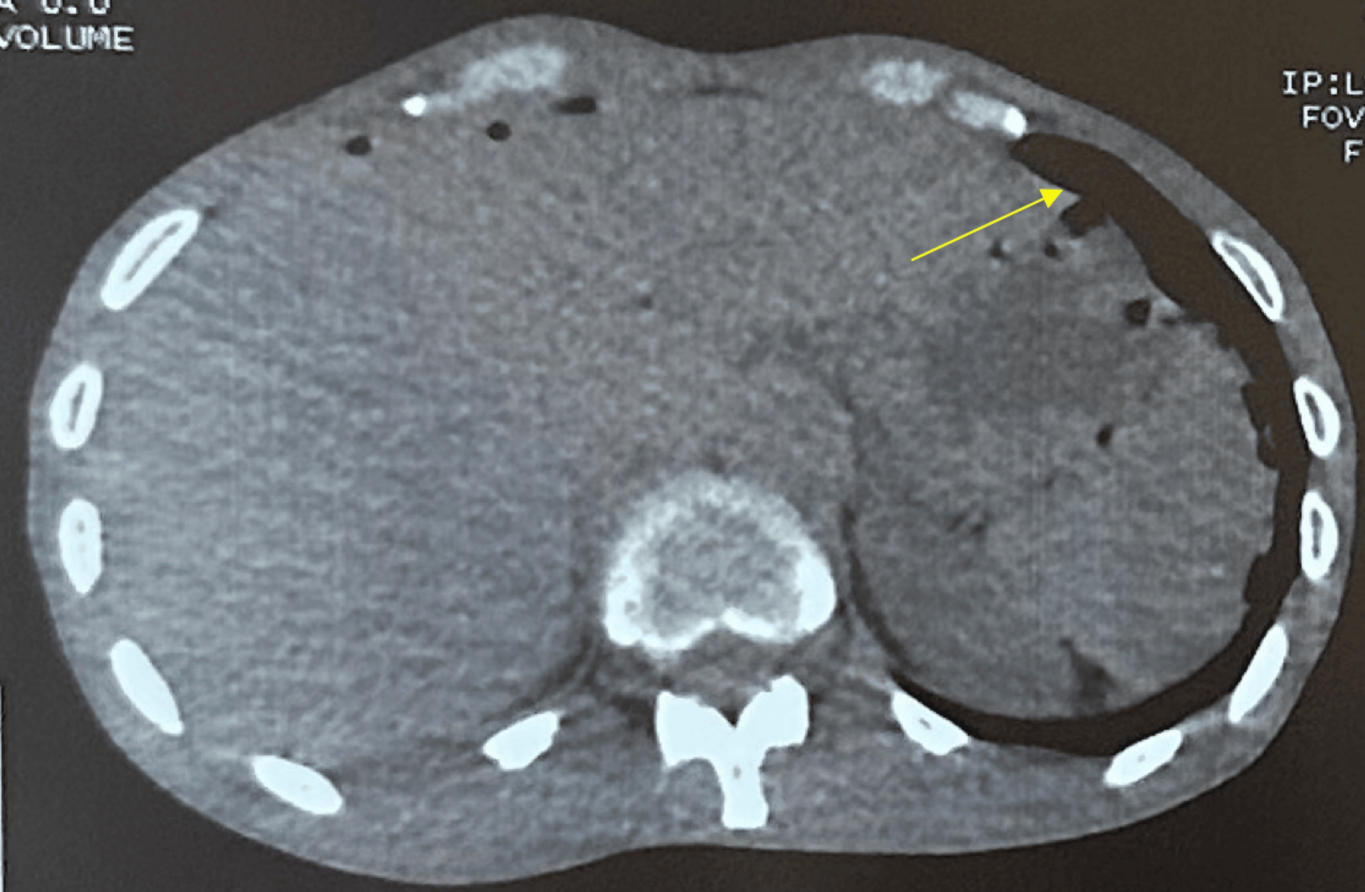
Abdominal CT scan showing pneumoperitoneum bubbles consistent with perforation of a hollow organ (arrow).
